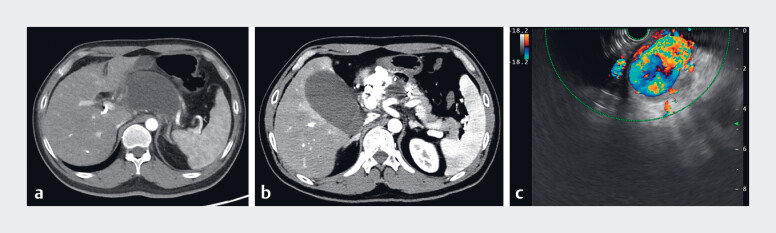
CT and endoscopic ultrasound (EUS) scan revealed the pseudocyst and residual arteriovenous fistula.
